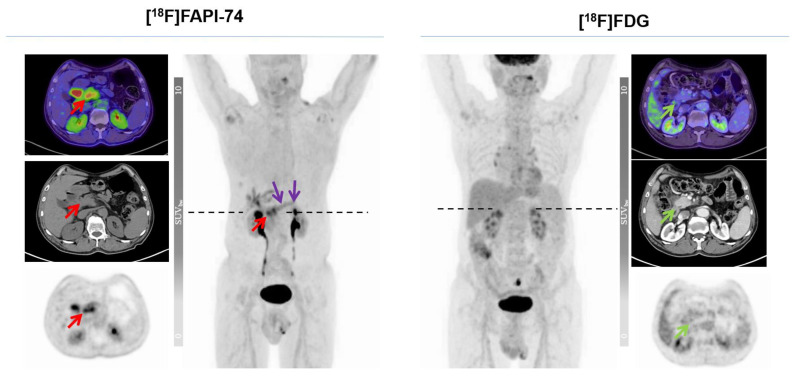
A 69-year-old male patient after neoadjuvant chemoradiotherapy in preoperative therapy response control with no significant uptake in [^18^F]FDG imaging. Red and green arrows indicate the primary lesion, while violet arrows address the confounding, clinically subtle, tumor-associated pancreatitis of the rest of the pancreas (SUV_max_ of the primary lesion in [^18^F]FDG and [^18^F]FAPI-74; 3.3 vs. 5.7, respectively).
